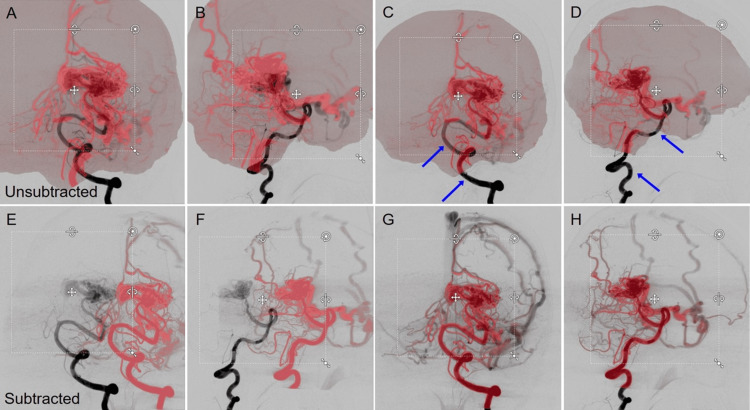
Comparison of Unsubtracted and Subtracted CBCTA Co-Registered with 2D-DSA for Left Occipital Arteriovenous Malformation. A–D: unsubtracted CBCTA; E–H: subtracted CBCTA; A, B, E, F: pre-fusion; C, D, G, H: post-fusion. Several defects on the major vessels (arrows) adjacent to the cranial bone are conspicuous on the unsubtracted CBCTA, whereas better vasculature visualization, including early filling veins, is demonstrated on the subtracted CBCTA.
